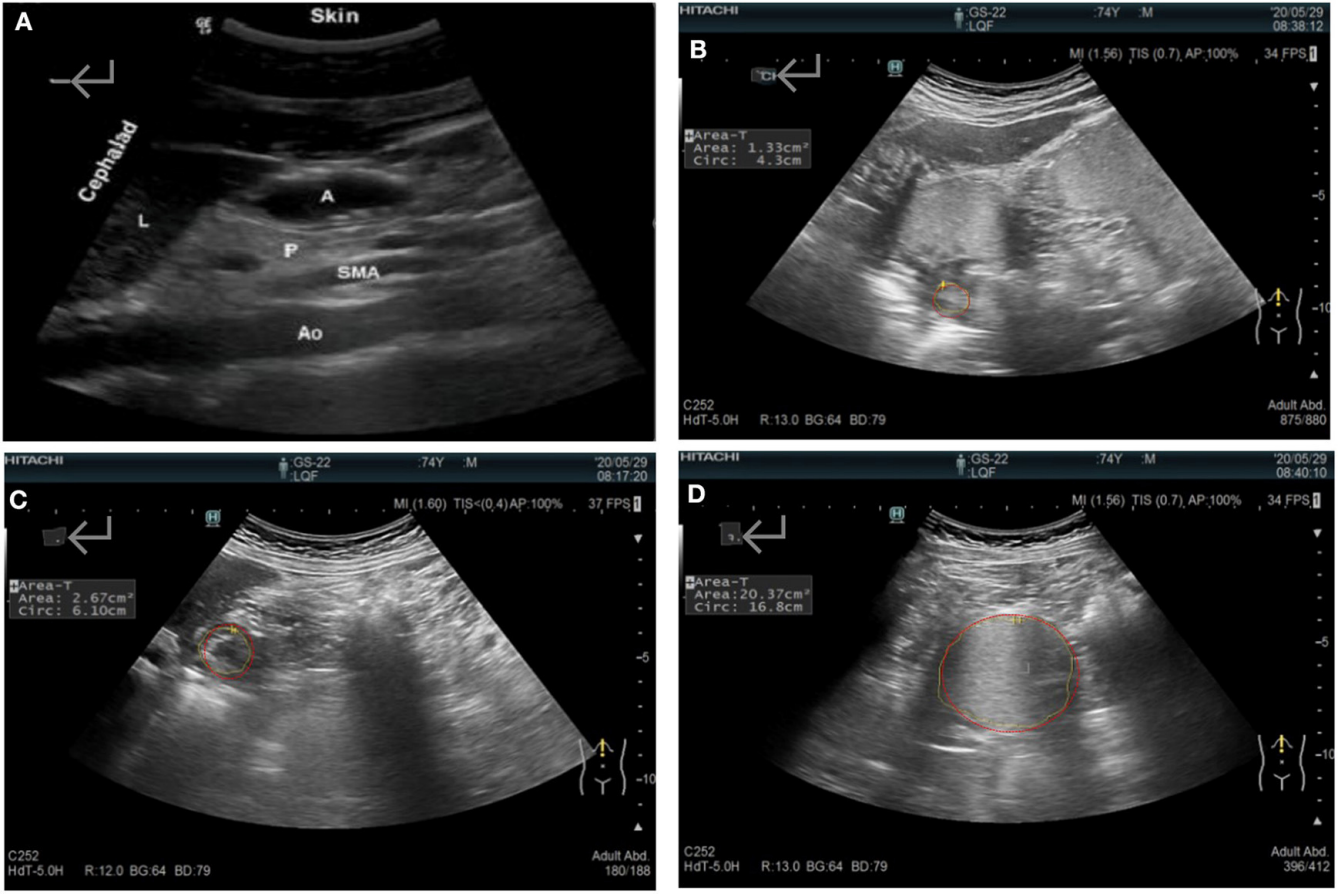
(A) Sonographic identification of the gastric antrum with the aid of anatomical landmarks: A, the gastric antral cross-sectional area; Ao, the abdominal aorta; L, the left lobe of the liver; P, the inferior pancreas; and SAM, the superior mesenteric artery. (B) The systolic antrum cross-sectional area. The gastric antrum cross-sectional area after (C) fasting and (D) immediately after a meal.
